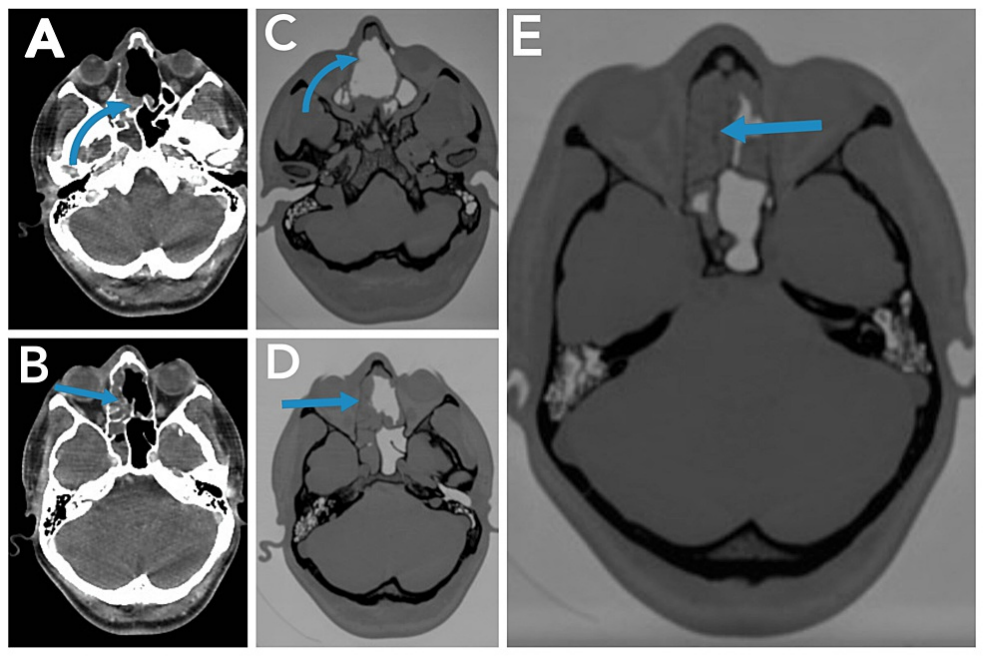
Axial sections of CT scan post-initial resection; the arrows in (A-E) show extensive post-surgical changes and/or residual disease involving the right maxillary antrum and nasal cavity
